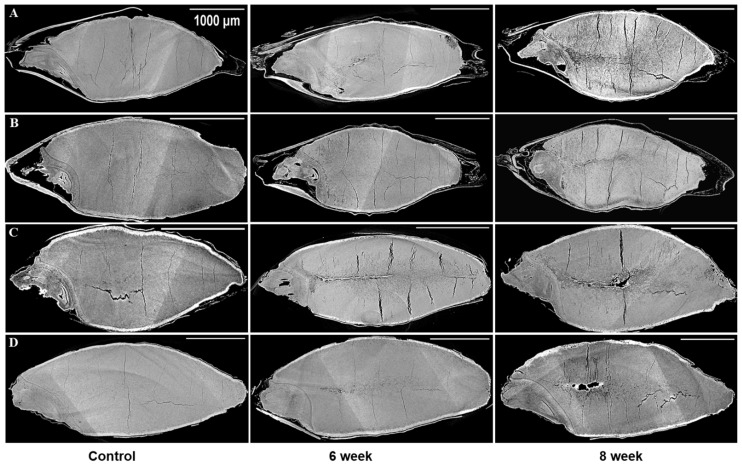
Longitudinal sections from 3D images of the control and 17% moisture content for four barley varieties stored for 6 and 8 weeks: (A) (6R) Tradition, (B) (2R) AC Metacalf, (C) (6Rhl) AB Cattlelac, (D) (2Rhl) Esma. The white bar in the X-ray projections represent the scalebar of 1000 µm.
